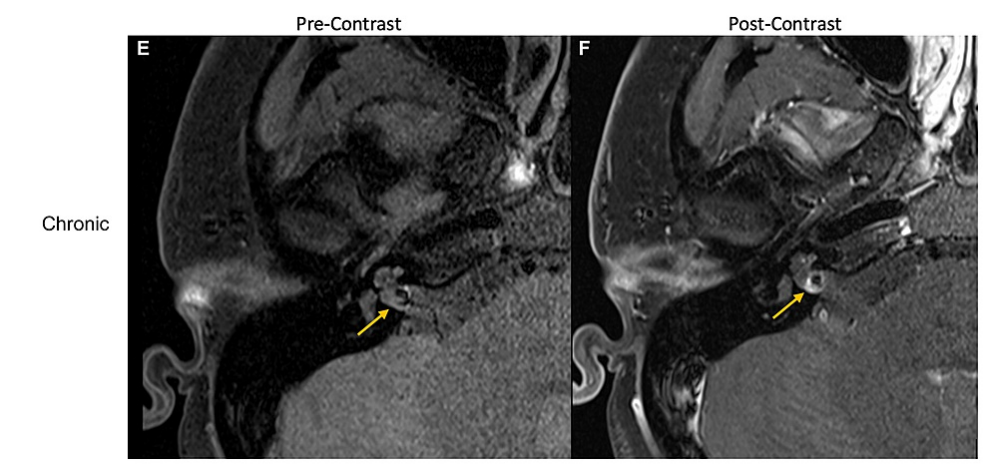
Right IAC lesion on MRI with and without contrast at chronic (two months after presentation) time point E: Pre-gadolinium T1-weighted axial MRI IAC showing an isointense IAC lesion with central hypointensity two months after the initial presentation F: Post-gadolinium T1-weighted axial MRI IAC showing the lesion as T1 hypointense with T1 intrinsic hyperintensity and with a surrounding margin of enhancement on postcontrast imaging, consistent with a chronic hemorrhage. These images follow the expected evolution of T1 precontrast appearance for blood products after an acute hemorrhage IAC: internal auditory canal; MRI: magnetic resonance imaging
